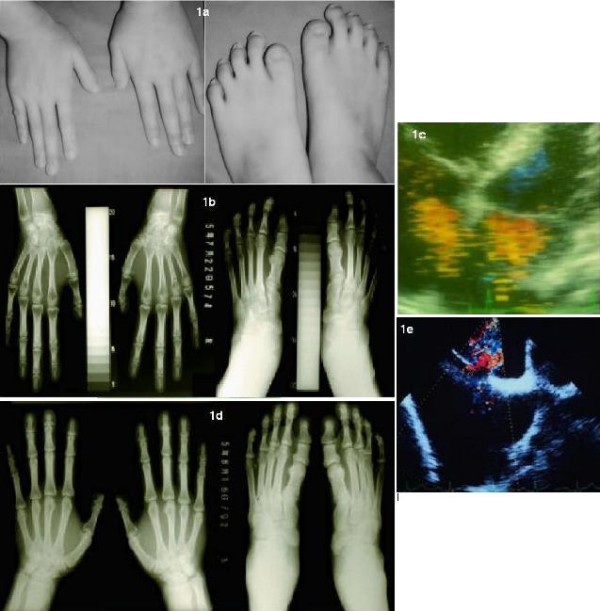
Patient's phenotype. (a) Photograph of both hands and feet of our patient. (b) Roentgenogram of the patient's hands and feet. (c) Echocardiogram of the patient (d) Roentgenogram of her father's hands and feet. (e) Echocardiogram of her father. Note symmetric hypoplasia of the first digit in both our patient and her father. Asterisk (*) displays ASD. RA, right atrium. LA, light atrium.
